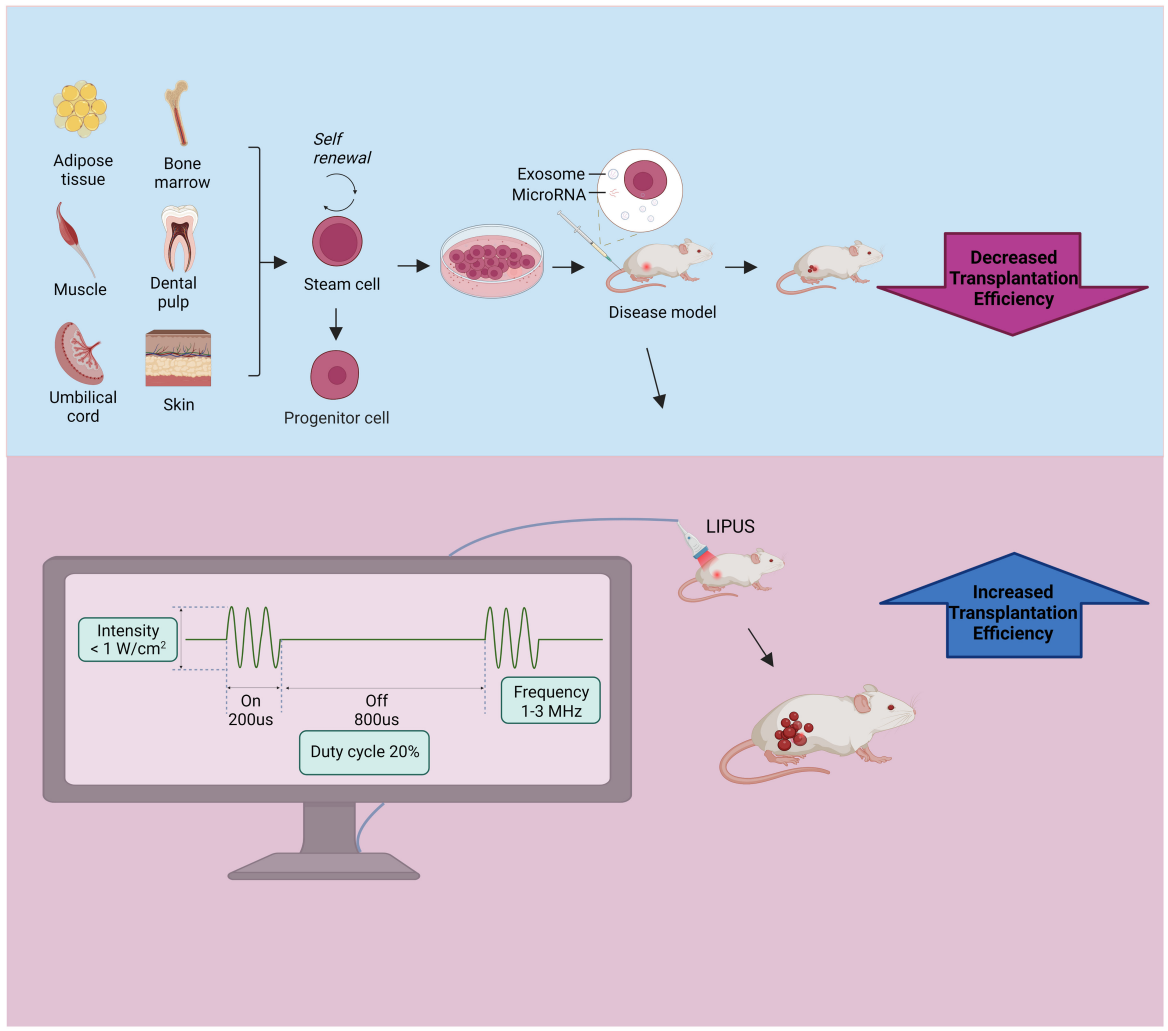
Stem cell and exosome injections are used to treat disease models, and further exposure to LIPUS can increase transplantation efficiency. The stem cell source is shown in the upper left, the lower left shows the common parameters of LIPUS, which features an intensity of less than 1 W/cm^2^, a frequency of 1-3 MHz, and a duty cycle of 20%. LIPUS, low-intensity pulsed ultrasound.
